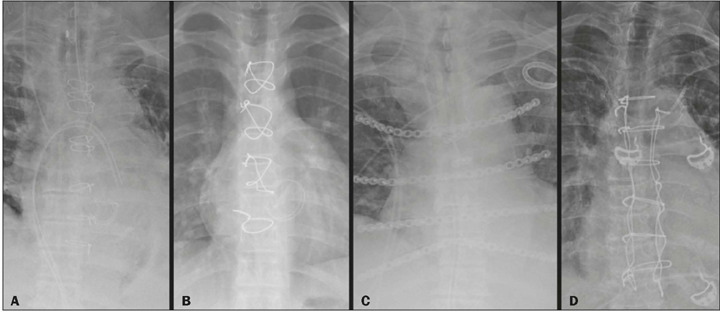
Sternum closure techniques (chest X-ray images). A:
